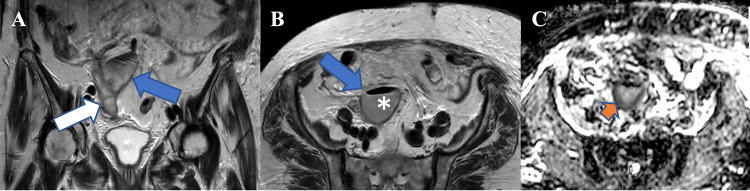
Coronal T2WI (A) shows a TOA (blue arrow) and a dilated tube filled with fluid (white arrow) in the projection of the right ovary, while axial T2WI (B) demonstrates the abscess (blue arrow) with a gas-fluid level (asterisk), and DWI image (C) reveals peripheral diffusion restriction (orange arrow)
